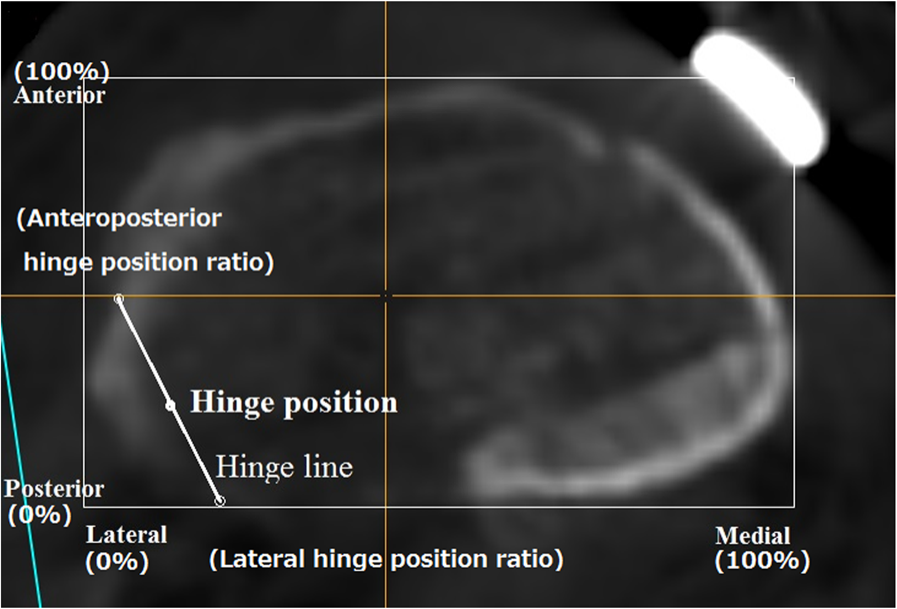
Cross-sectional computed tomography image on the upper osteotomy plane. In the upper osteotomy plane, the midpoint of the line connecting the two points where the hinge line intersects the proximal tibial lateral cortex (hinge position) is displayed on the x-y coordinate system based on the mediolateral and anteroposterior width
